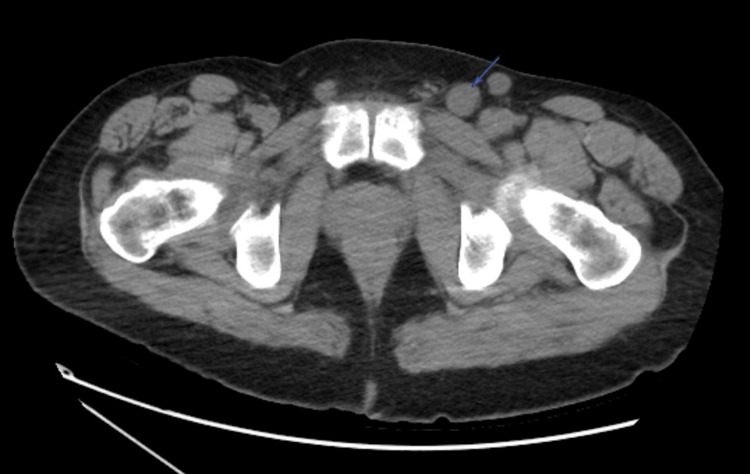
Axial CT scan of the pelvis without intravenous contrast revealing left inguinal lymphadenopathy (blue arrow) CT: computed tomography
